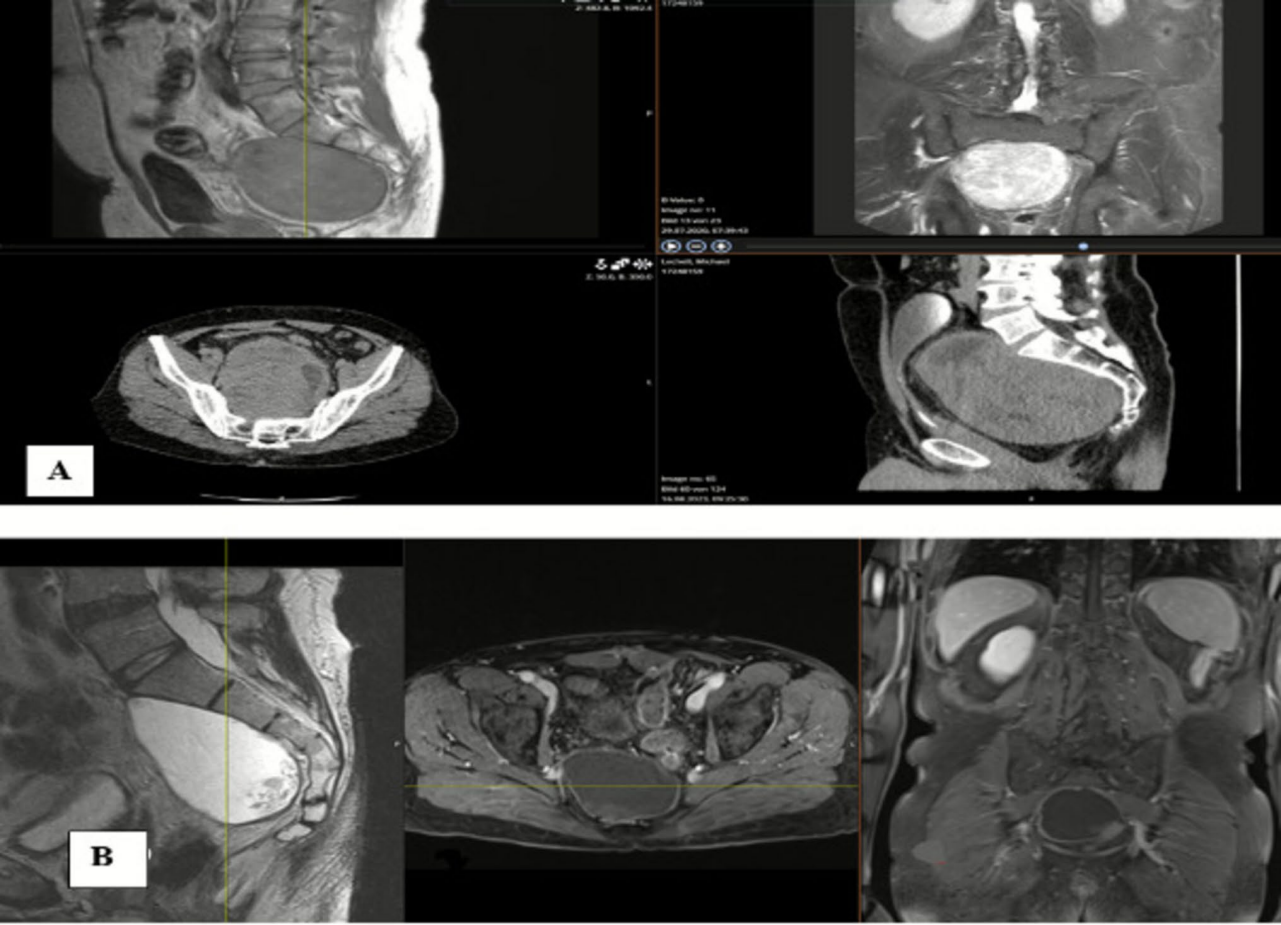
A CT and MRI showing Contrast enhanced large presacral lasion (16 × 14 × 12 cm) extending into the lesser pelvis. B Postoperative contrast-enhanced MRI demonstrated a fluid collection within the resection cavity, with no contrast uptake or residual tumor
